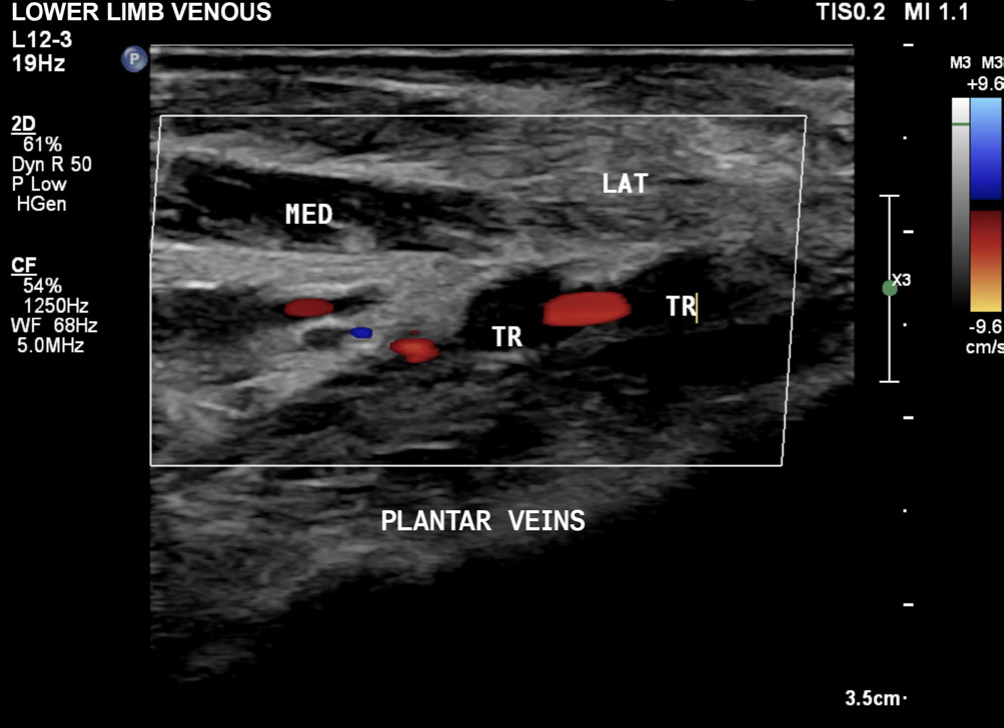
Plantar vascular ultrasound of the right lower limb, in the transverse plane, showing venous thrombosis of the lateral plantar vein. TR = thrombus; MED = medial compartment; LAT = lateral compartment.
